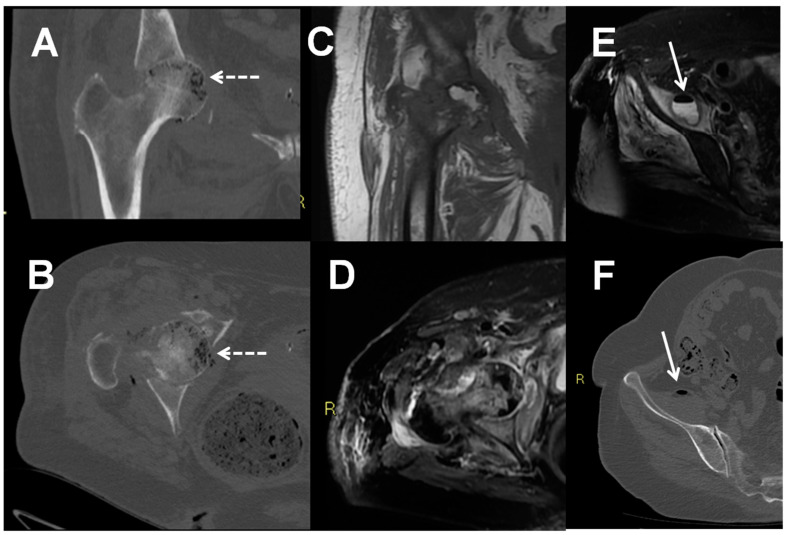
Septic arthritis of the right hip in a 78 years old woman with studied with x-rays (A), CT (B,F), and MRI (C,D,E). CT showed bone intramedullary air coefficients (broken arrows) and involvement of the homolateral ileo-psoas muscle (arrows).
